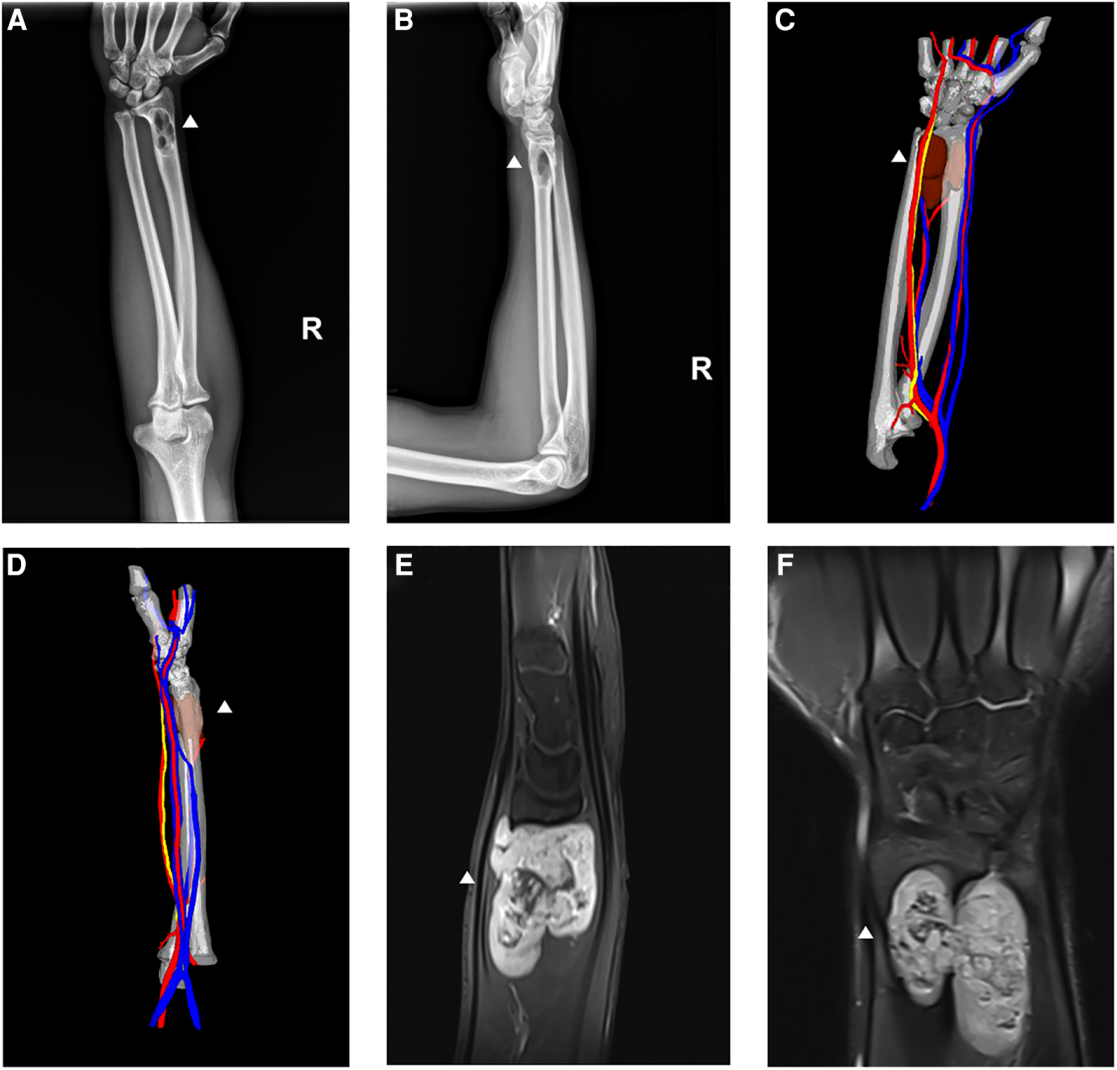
Preoperative imaging evaluation of the right forearm (white triangle indicates the location of the lesion). (A,B) Preoperative anteroposterior and lateral radiographs revealing the lesion in the right radius. (C,D) Three-dimensional computed tomography reconstruction demonstrating the positional relationship between the tumor and the bone in the anteroposterior and lateral views. (E,F) Lateral and anteroposterior magnetic resonance imaging indicating the invasion. The lesion measured approximately 4.8 cm × 3.7 cm × 3.6 cm, inclusive of the occupied radius.
